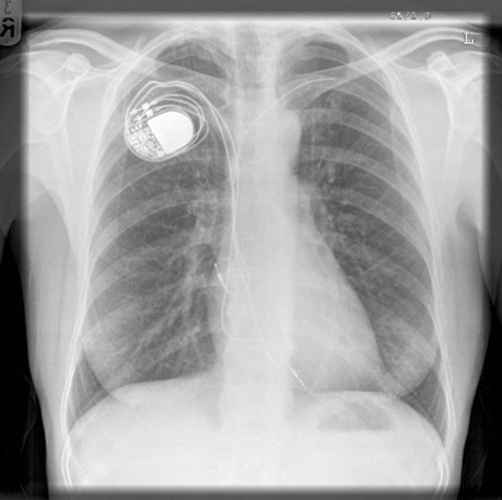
Chest X-ray showing pacemaker and pacing leads.
